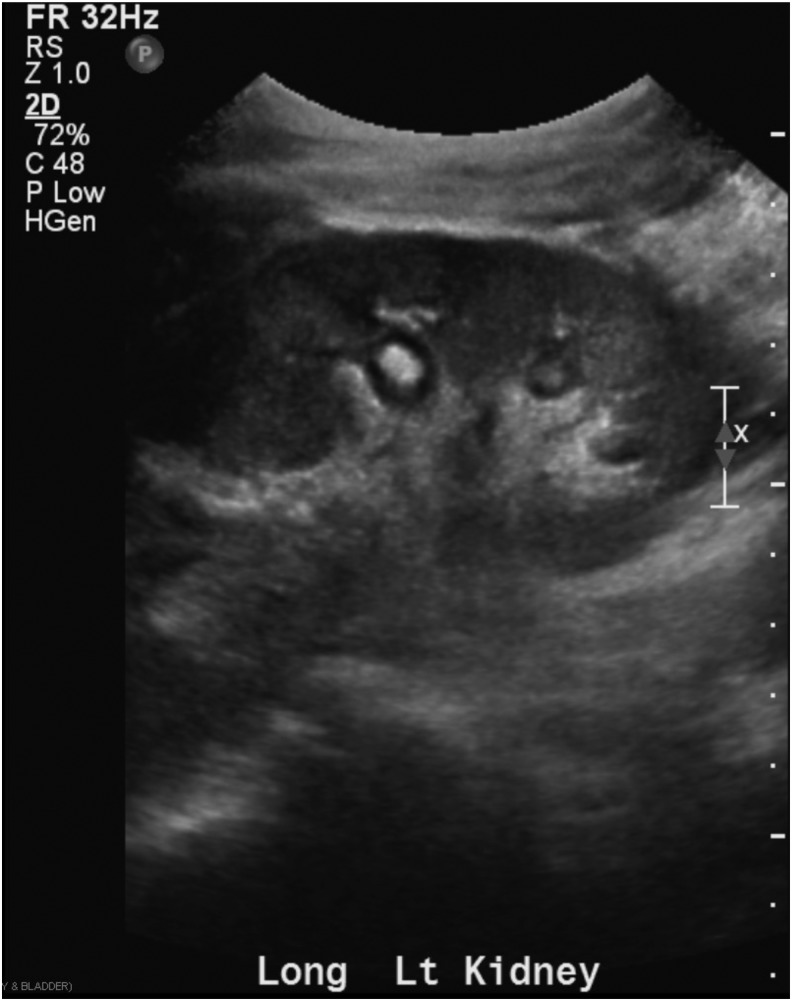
Renal ultrasonography. Seven millimeter, left sided interpolar nonshadowing hyperechoic foci in the renal collecting system.
